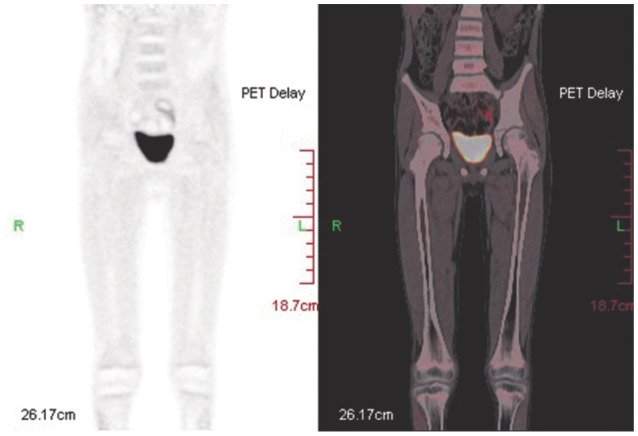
患者2022年7月4日局部PET-CT：骨盆左半部分及双侧股骨下段及胫腓骨上段部分代谢稍活跃
